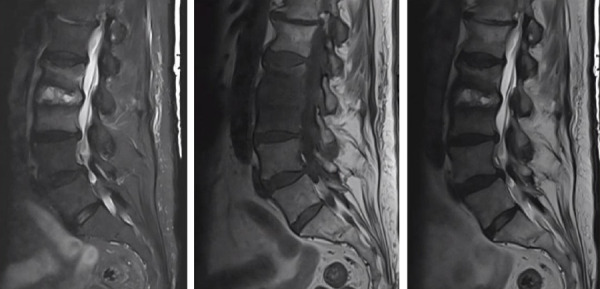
MRI of the lumbar spine which shows infiltrative T1 marrow signal involving L2 and L3 vertebral bodies with associated postcontrast enhancement. Fluid/edema within the L2-L3 disc space.
